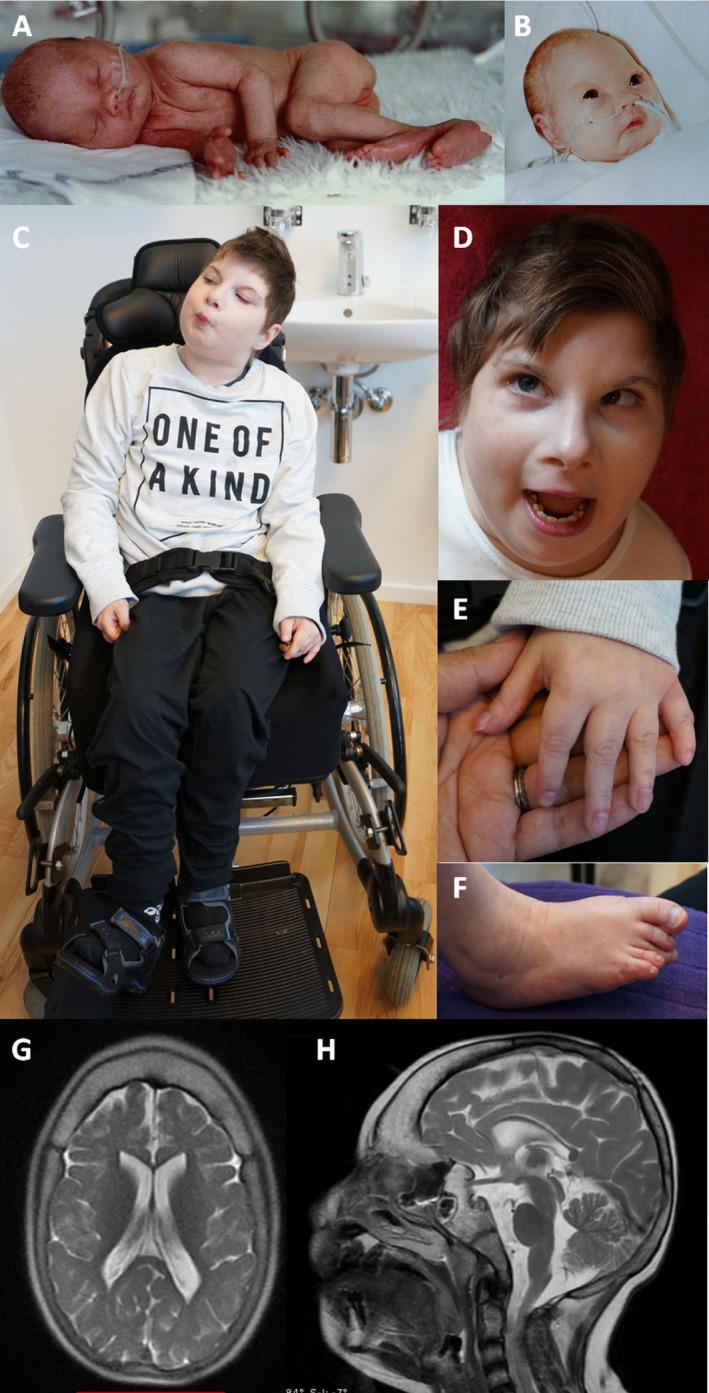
Clinical photographs showing dysmorphic features of the two siblings. (A) and (B) Older sister as a newborn: microcephaly, hypertelorism, upslanted palpebral fissures, flat nasal bridge, downturned corners of the mouth, micrognathia, fifth finger clinodactyly, rocker‐bottom feet. Index patient aged 22 years (C) Full body image (D) Craniofacial features: microcephaly, convergent squint, hypoplastic alae nasi, micrognathia (E) Small hands with tapering fingers and fifth digit clinodactyly (F) Right foot showing overlapping toes and hypoplastic toe nails (G) and (H) MRI brain (index patient): Axial and sagittal sections showing microcephaly and thickening of frontal and sphenoid bones skull. Cortical patterning and intracranial structures appear normal.
